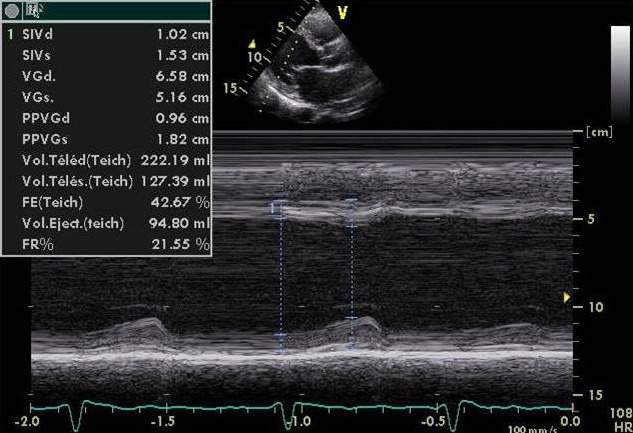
Coupe TM sur le VG en incidence parasternale grand axe montrant une dilatation et une dysfonction systolique ventriculaire gauche
